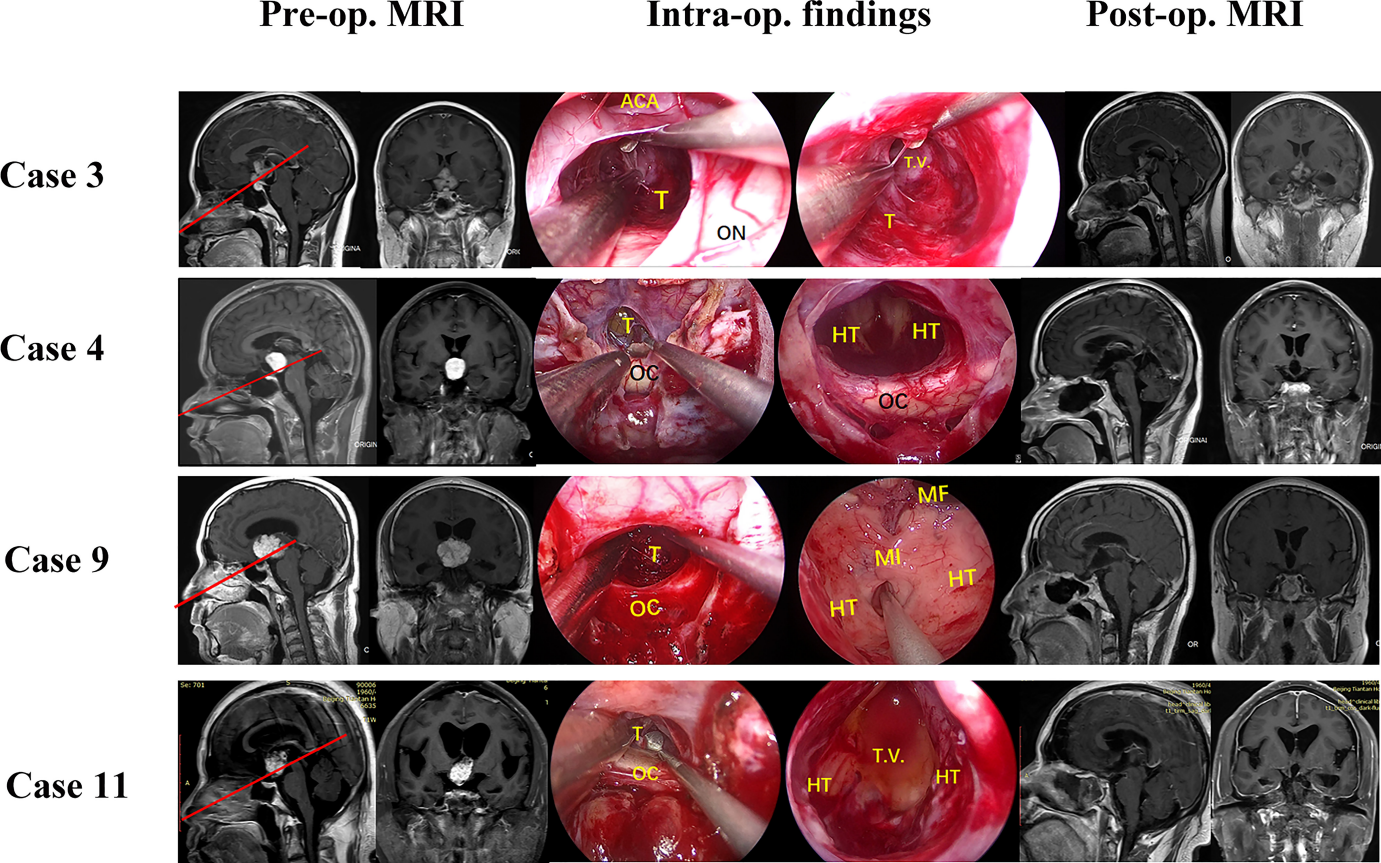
Pre- and post-operative MRI and intraoperative findings of 11 patients treat by combined trans-lamina terminalis and trans-pituitary-chiasm corridors. Case 3, a germinoma with supra-NCL part about 80% were partial resected *via* a TLTA and a radiotherapy and chemotherapy were followed. Case 4, a chordoid glioma with supra-NCL part about 95% were total removed by TLTA. Case 9, a papillary craniopharyngioma with supra-NCL part about 95% were removed totally by TLTA. Case 11, a papillary craniopharyngioma with supra-NCL part about 90% were removed totally by TLTA. OC, optic chiasm; PS, pituitary stalk; ON, optic nerve; T, tumor; P, pituitary; ICA, internal carotid artery; T.V., 3^rd^ ventricle; LT, lamina terminalis; HT, hypothalamus; MF, Monro’s foramen; TLTA,trans-lamina terminalis approach.
